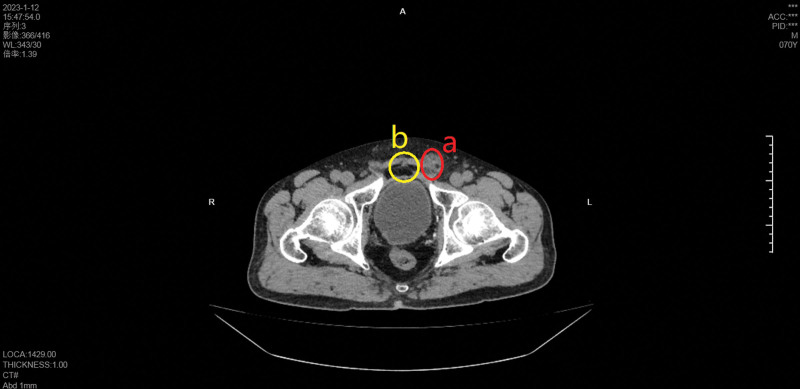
Computed tomography (CT). (A) The left inguinal hernia. (B) The scar of laparoscopic radical prostatectomy.
